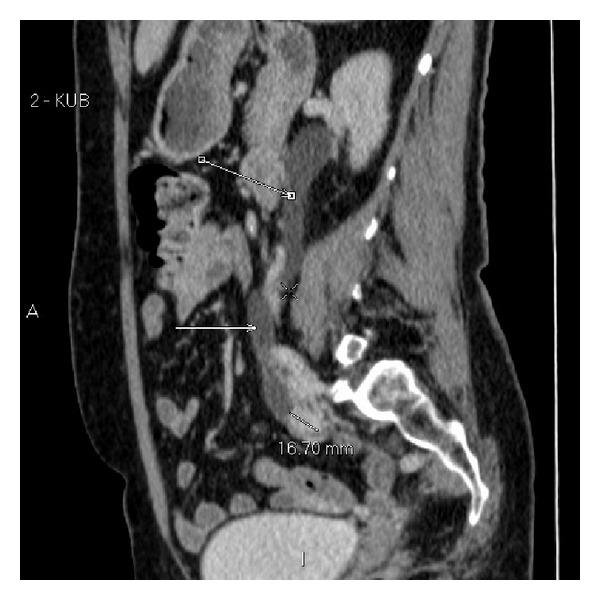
Portal venous phase (split bolus) CT urogram at time of staging. This shows an obstructing, synchronous, enhancing 16 mm soft tissue lesion in the lower third of the right ureter. Arrows demonstrate hydroureter above obstruction.
